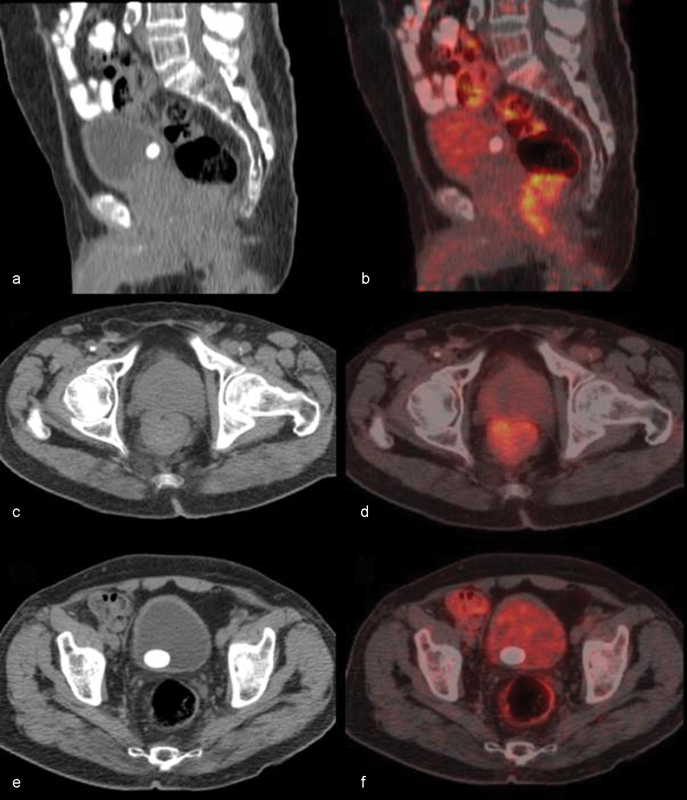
The F-18 FDG-PET/CT revealed primary rectal tumoral lesion, and regional lymph nodes were FDG (−). (a) Sagital CT, (b) Sagital fusion, (c) Axial CT and (d) Axial fusion images of the primary hipermetabolic rectal malign melanoma (e) Axial CT and (f) Axial fusion images of milimetric FDG(−) pelvic lymph nodes.
